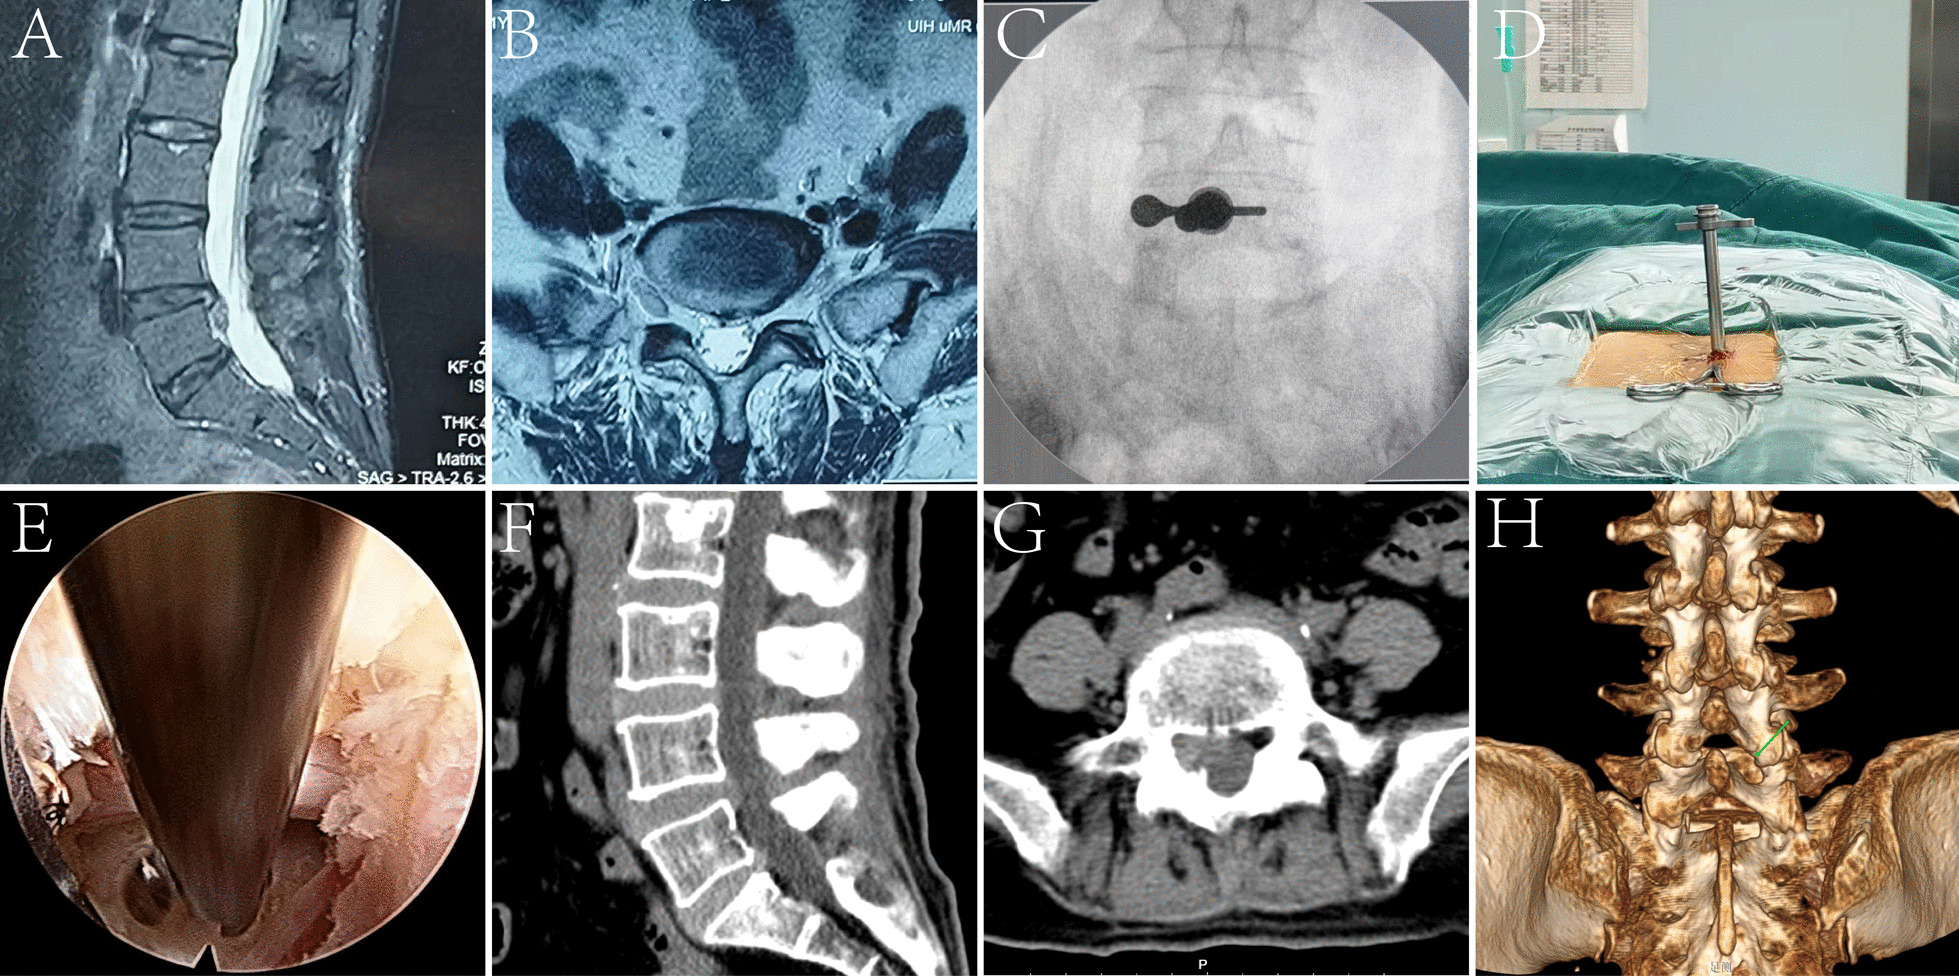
Surgical procedure of PEID and pre/postoperative imaging of patients with high-grade downward migrated LDH. A, B Preoperative sagittal and transverse MRI showed high-grade downward migration of the L4/5. C–E Image C showed the anteroposterior fluoroscopy utilized during the PEID procedure. Image D showed the PEID working channel. Image E demonstrated the decompressed nerve root following PEID. F–H Postoperative sagittal and coronal CT imaging of the operated area reveals the absence of any remaining migrated nucleus pulposus. Additionally, 3D reconstruction enables clear visualization of the defective bone
